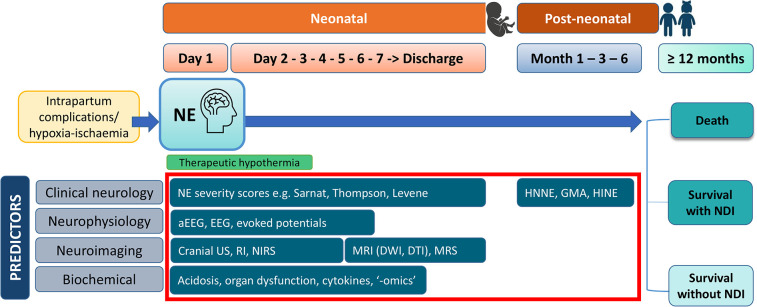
Conceptual framework for the predictors of adverse early childhood outcome following intrapartum-related neonatal encephalopathy. This figure displays predictors that will be specifically evaluated for in this review, within the red box. aEEG, amplitude-integrated encephalography; DTI, diffusion-tensor imaging; DWI, diffusion-weighted imaging; EEG, electroencephalography; GMA, Prechtl's general movements assessment; HINE, Hammersmith Infant Neurological Examination; HNNE, Hammersmith Neonatal Neurological Examination; MRI, magnetic resonance imaging; MRS, magnetic resonance spectroscopy; NDI, neurodevelopmental impairment; NE, intrapartum-related neonatal encephalopathy; NIRS, near-infrared spectroscopy; RI, resistive index; US, ultrasound.
